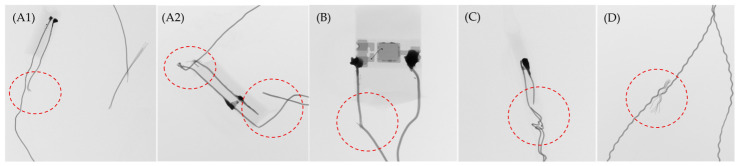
X-ray images of different E-yarn types after failure under quasi-static tensile loading, with failure locations indicated by red circles in each. (A1) End-soldered LED E-yarn. (A2) Mid-soldered LED E-yarn. (B) End-soldered PD E-yarn. (C) End-soldered resistor E-yarn. (D) Braided Litz wires.
